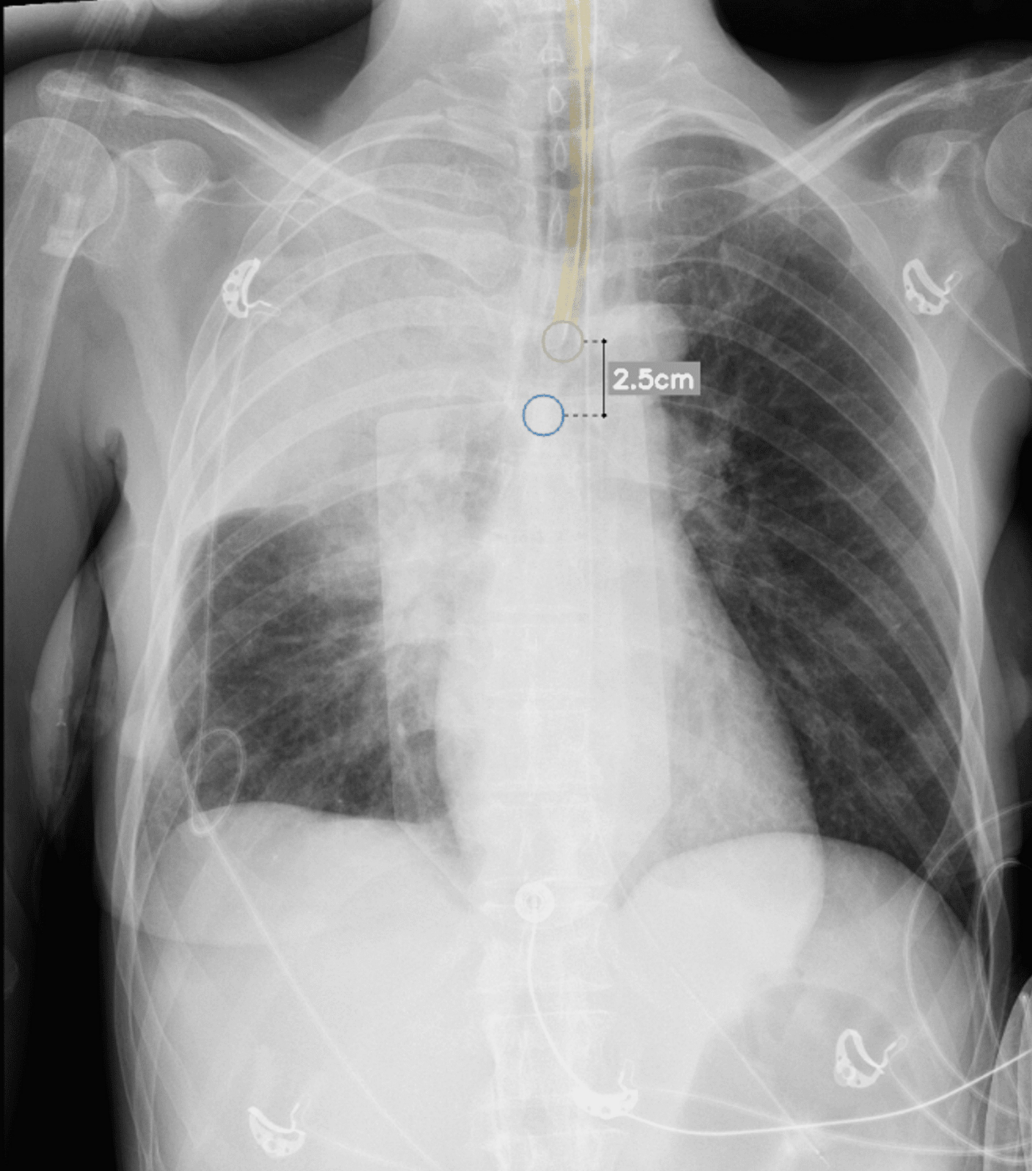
Chest radiograph obtained immediately after endotracheal intubation (pre-bronchoscopy) revealing consolidation/complete collapse of the right upper lobe.
